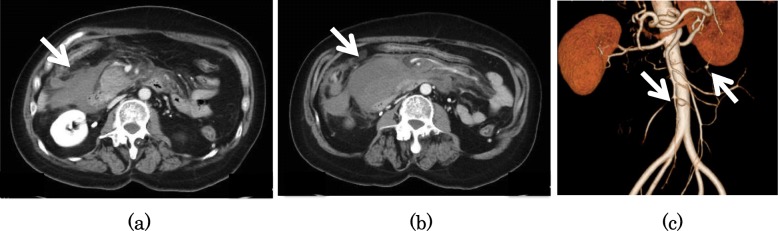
Enhanced abdominal computed tomography showed a hematoma in the retroperitoneum (a), a lesion suggestive of an aneurysm in the head of the pancreas (b), and multiple aneurysms arising from the jejunal artery (c)
